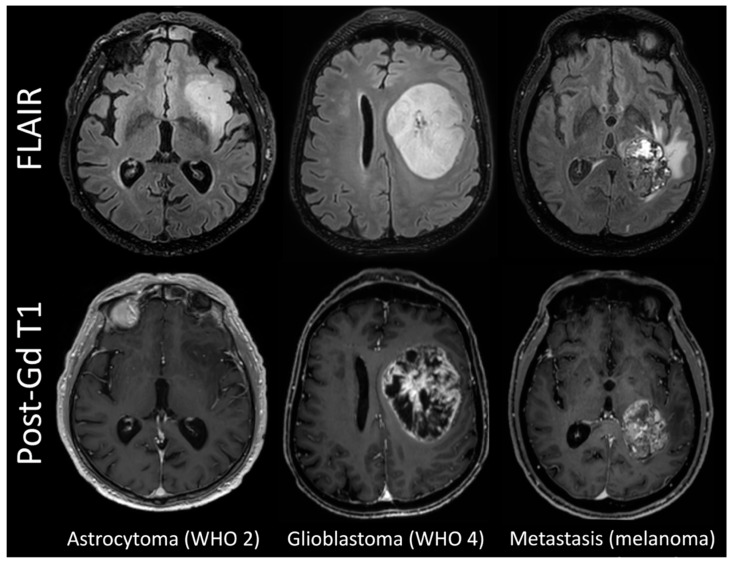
FLAIR and post-contrast T1w images in representative cases.
